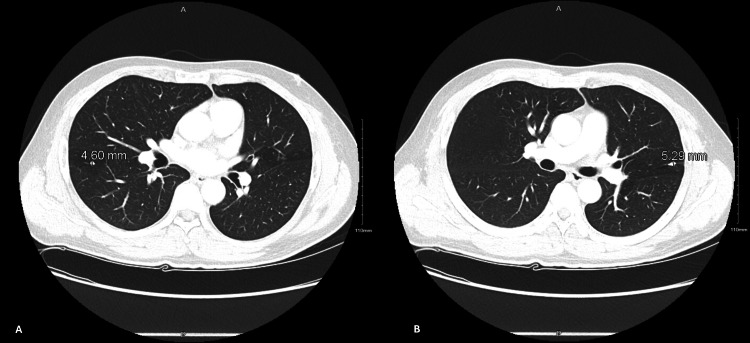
Contrast-enhanced chest CT demonstrating a 4.60 mm calcified nodule in the superior segment of the right upper lobe (A) and a 5.29 mm calcified granuloma in the lingular segment of the left upper lobe (B), both consistent with healed granulomatous infection.
